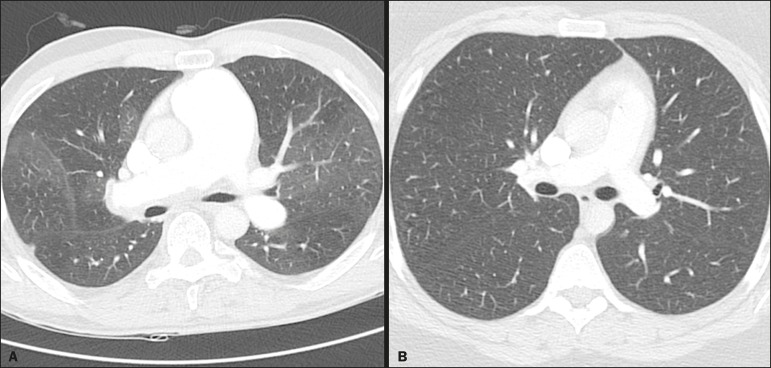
Axial CT images showing the difference in pulmonary attenuation between a
